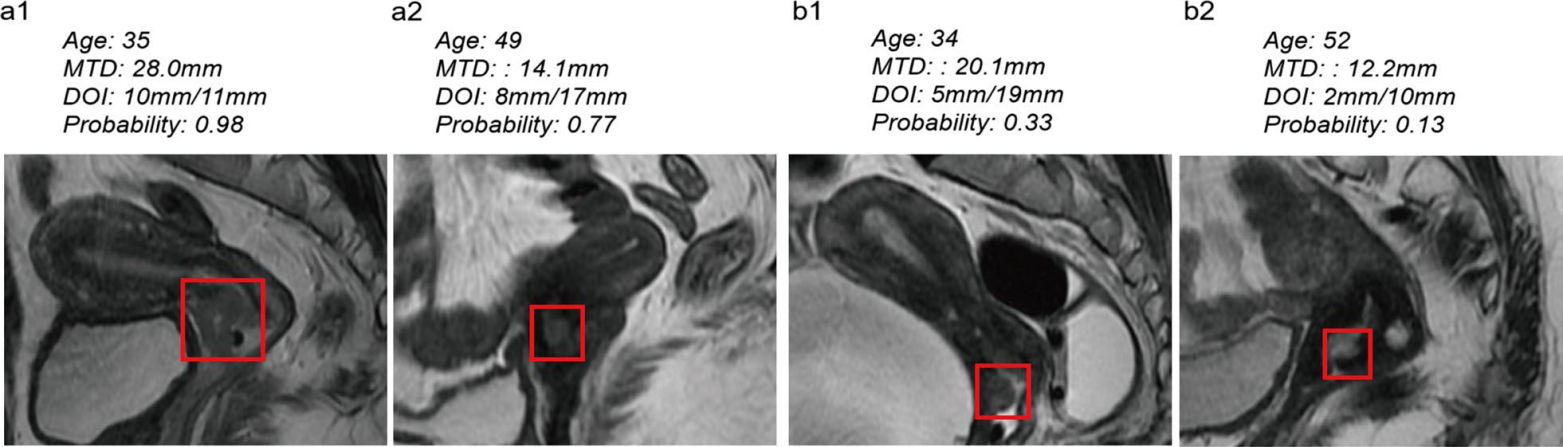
Representative images of middle or deep cervical stroma invasion (a) and superficial cervical stroma invasion (b). The lesions in the frames on sagittal T2WI are cervical tumors. a1 a 35-year-old, 2018 FIGO IB2, SCC patient with MTD on MRI of 28.0 mm. The probability of the middle or deep stroma invasion predicted by the nomogram was 98%. a2 a 49-year-old, 2018 FIGO IB1, SCC patient with MTD on MRI of 14.1 mm. The probability of the middle or deep stroma invasion predicted by the nomogram was 77%. b1 a 34-year-old, 2018 FIGO IB2, SCC patient with MTD on MRI of 20.1 mm. The probability of the middle or deep stroma invasion predicted by the nomogram was 33%. b2 a 52-year-old, 2018 FIGO IB1, AC patient with MTD on MRI of 12.2 mm. The probability of the middle or deep stroma invasion predicted by the nomogram was 13%. (MTD: maximal tumor diameter; FIGO: Federation International of Gynecology and Obstetrics; SCC: squamous cell carcinoma; AC: adenocarcinoma)
